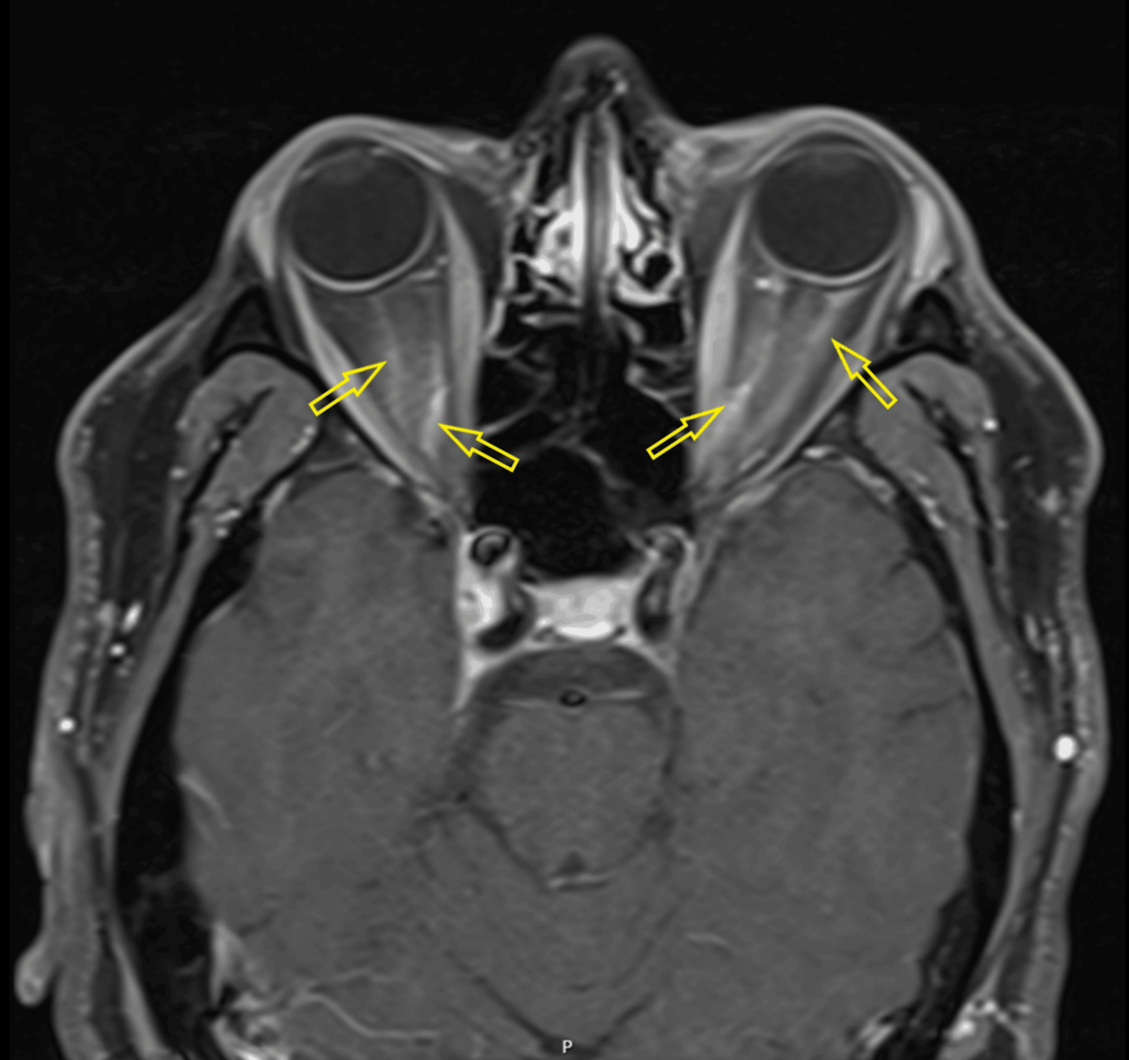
Post-contrast T1-weighted axial MRI demonstrating the contrast enhancement of the optic nerve sheath bilaterally.
